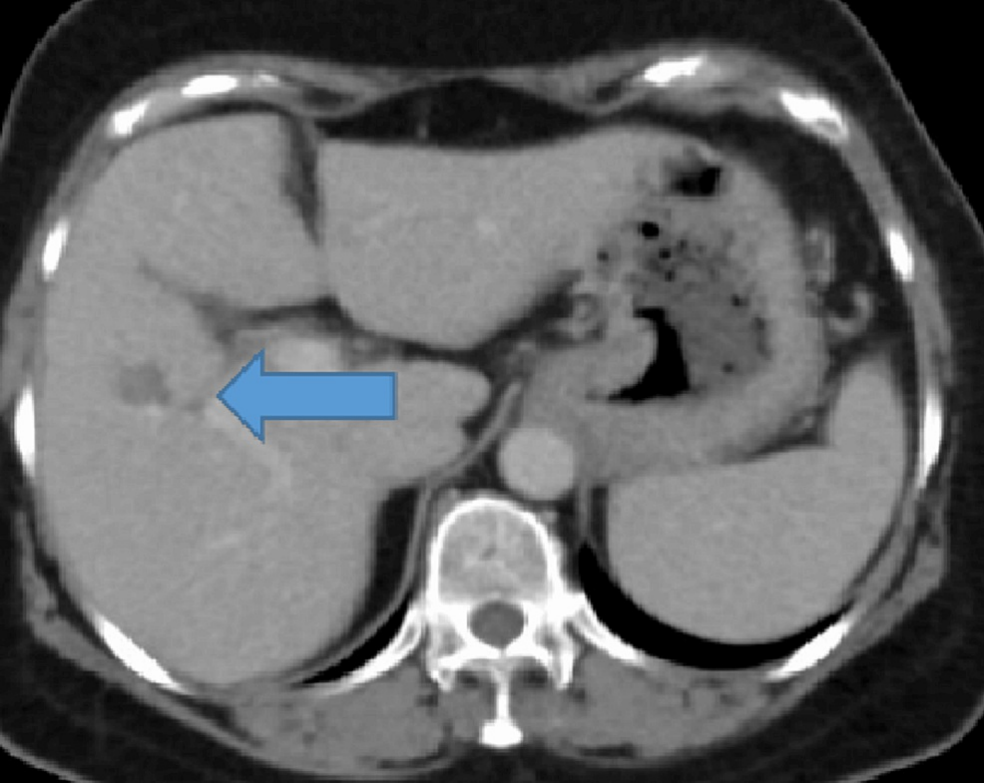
Axial view of CT of the chest, abdomen, and pelvis (CAP) showing satisfactory post-radioablative changes in segment 5 of the liver.
